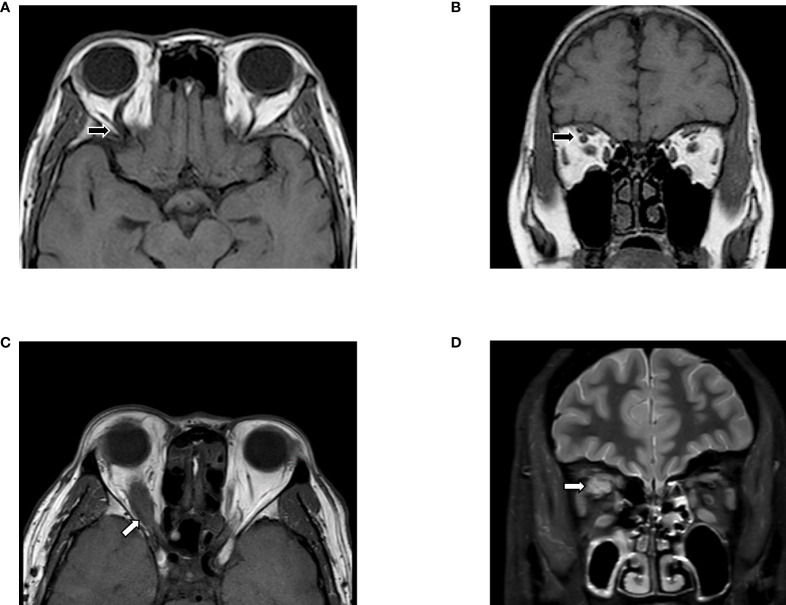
Differential diagnosis based on MRI. (A, B) Slightly enlarged EOMs were shown in T1WI, with the unilateral, augmented superior ophthalmic vein (black arrows). Features indicated carotid-cavernous fistulas instead of GO, which need to confirm *via* digital subtraction angiography (DSA). (C, D) MRI images suggested Imbalanced exophthalmos and the apparent swelling of superior rectus in the right eye. Meanwhile, in T2FS, increased signal of EOMs, combined with the tendon involved (white arrows) enlargement in superior rectus suggested orbital myositis. The figure is original.
